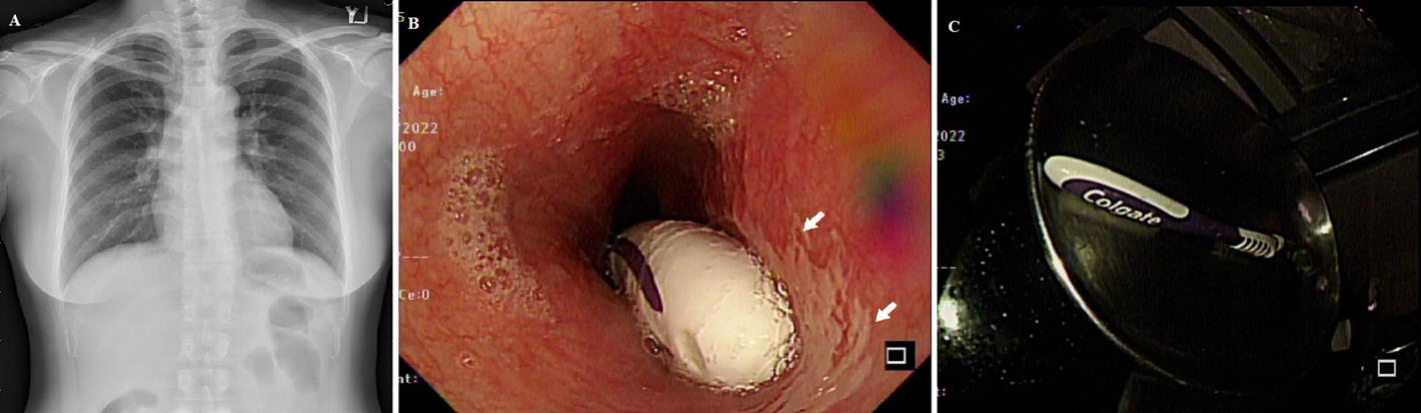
A) chest X-ray showing no evidence of toothbrush; B) toothbrush impacting at lower esophagus with ulcer formation; C) the 18cm impacting toothbrush was removed from the esophagus endoscopically
